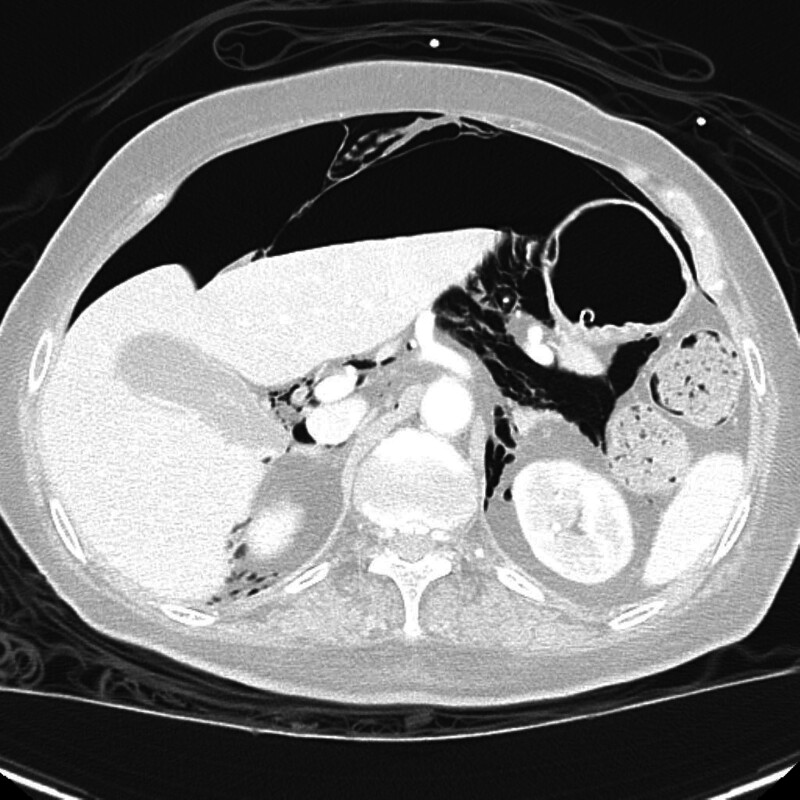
Axial CT at the lower thoracic level shows significant bilateral pneumothorax and diaphragmatic outline elevation. The left lung is markedly collapsed, and a large air pocket is visualized within the left pleural space. The diaphragm appears sharply outlined by subdiaphragmatic free air, supporting the presence of associated pneumoperitoneum. CT = computed tomography.
